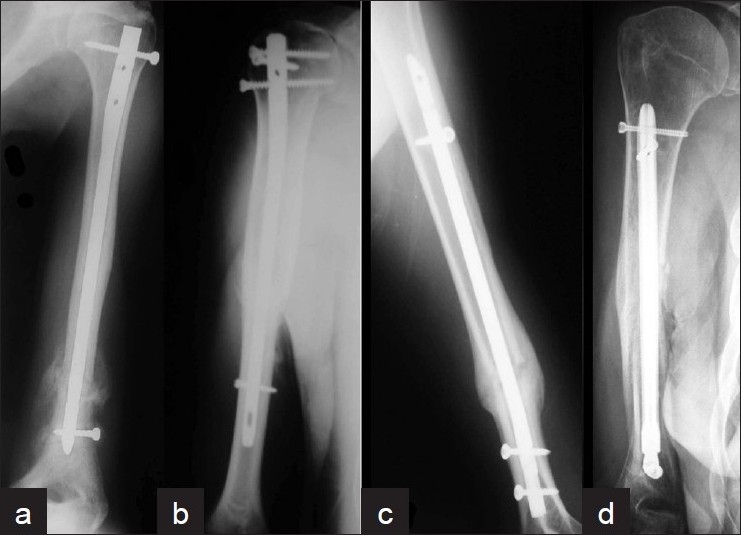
Plain radiographs of arm of “Fixed” nails (anteroposterior view) showing (a) Uniflex (Biomet), (b) Polarus (Acumed), (c) UHN (Synthes), (d) Garnavos (MERETE)
